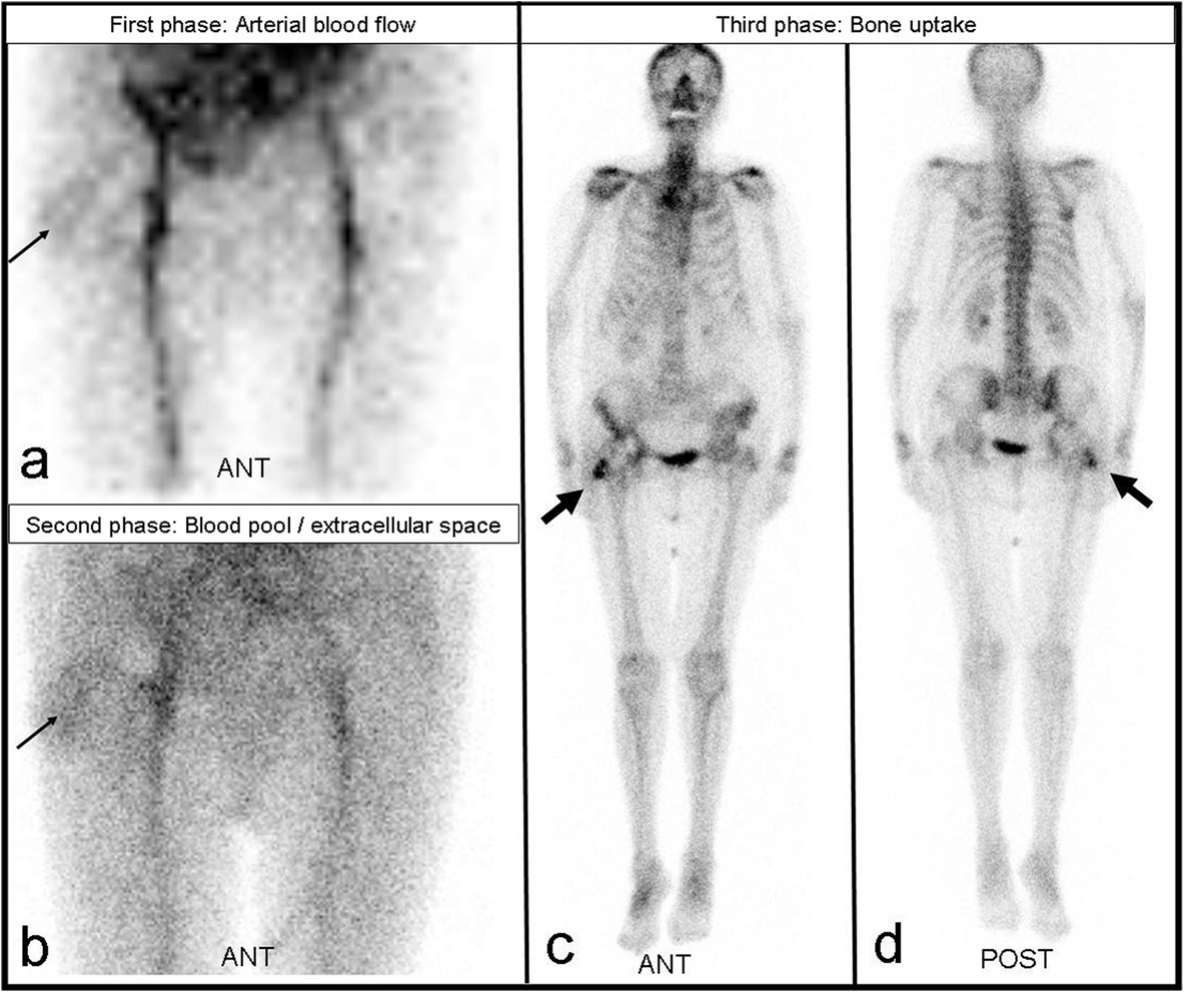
Three-phase bone scintigraphy. [99mTc] DPD three-phase bone scintigraphy. Increased blood flow (a) and blood pool (b) in the soft tissue of the right hip, lateral of the total hip arthroplasty (thin arrows) as well as increased bone uptake (c, d) in the lateral parts of the right hip (bold arrows) in a patient with infection of the right total hip arthroplasty
